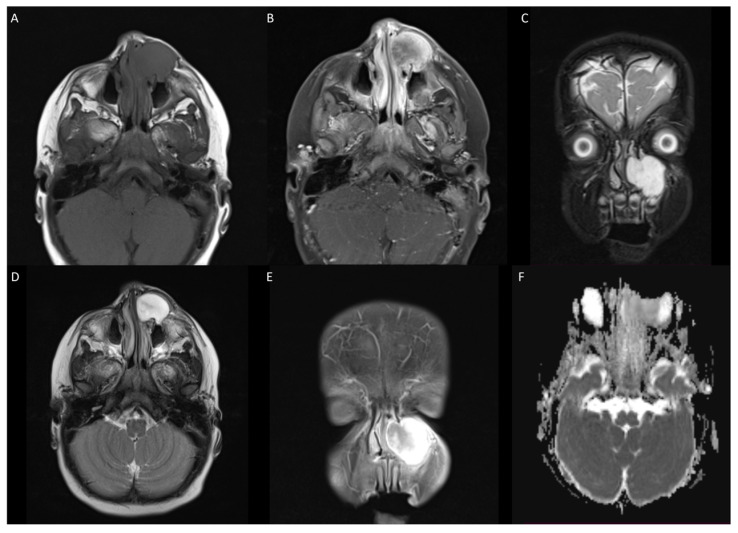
(A) T1 weighted image showing hypointense lesion in left maxilla extending infraorbitally. (B,E) T1 with contrast showing avid heterogenous enhancement of lesion. (C) T2 coronal demonstrating superior and inferior extension of lesion. (D) T2 fat-suppressed axial with hyperintense lesion. (F) DWI showing no apparent hyperintensity or low ADC values.
